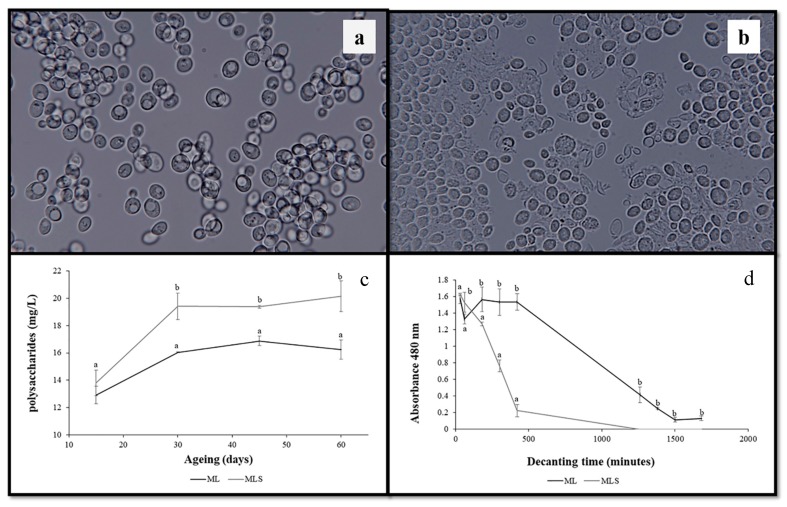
(a) Wet lees without ultrasound treatment; (b) wet lees after twenty minutes of ultrasound treatment; (c) evolution of the polysaccharides content (mg/L) in hydroalcoholic solutions, throughout the Ageing on Lees (AOL); (d) decanting time measured by spectrophotometry, at 480 nm (absorbance units). ML—hydroalcoholic solution with lees; MLS—hydroalcoholic solution with sonicated lees. Mean ± standard deviation of the three replicates. Different letters in axes X in figures (c) and (d) indicate values with statistical significant differences (*p* < 0.05).
